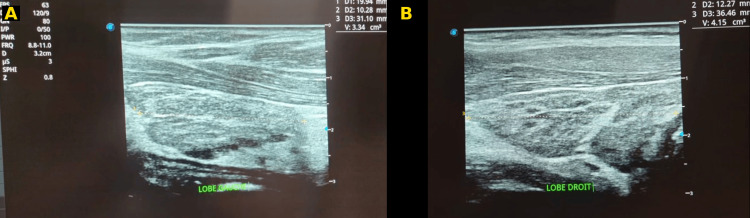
Thyroid ultrasonography showing bilateral gland atrophy with heterogeneous echotexture. Gray-scale ultrasound images showing reduced thyroid volume and diffuse heterogeneous echotexture involving both lobes. (A) Left thyroid lobe (volume 3.34 cm³). (B) Right thyroid lobe (volume 4.15 cm³).
